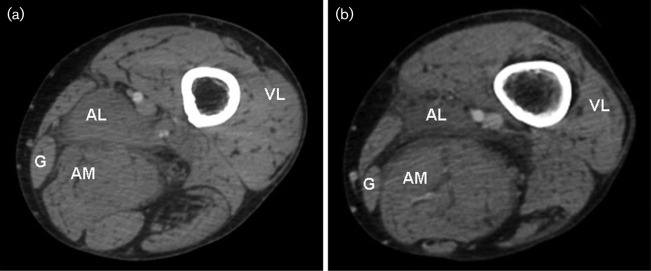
Radiological findings. (a, b) Axial CT sections through the left thigh following intravenous contrast at initial presentation with thigh pain. The posterior compartment, adductor longus (AL) and magnus (AM), gracilis (G) and vastus lateralis (VL) are visualized, as are the femoral artery and vein (in the adductor canal, medial and superficial to AL). CT examination noted increased oedema and heterogeneous density within AL and adjacent inflammatory stranding (b).
